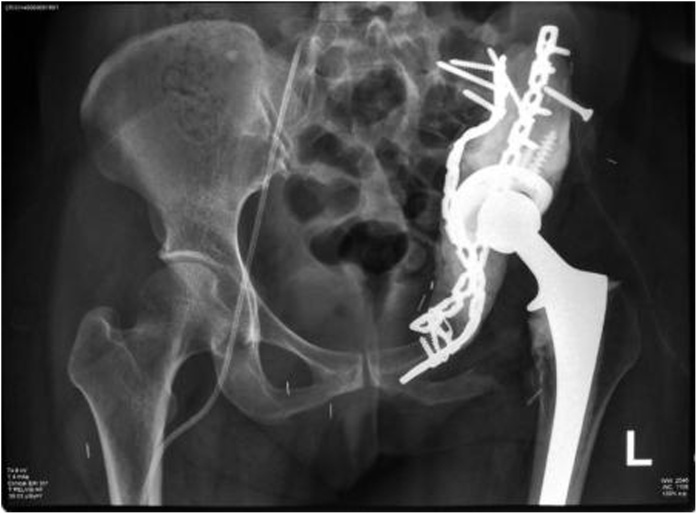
Postoperative X-Ray Imaging Showing Plate, Screw, and Total Hip Replacement.
